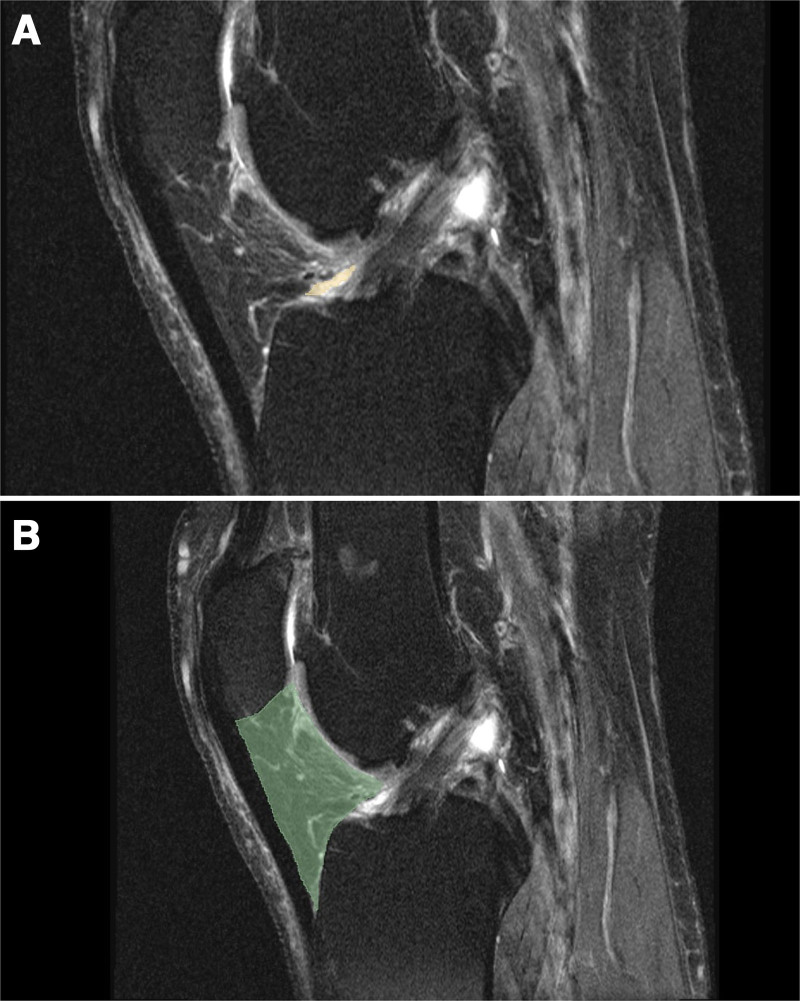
(A) Sagittal MRI section of a patient. (B) The boundaries of Hoffa fat pad in the sagittal MRI section were identified through segmentation using the 3D Slicer Software. MRI = magnetic resonance imaging.
